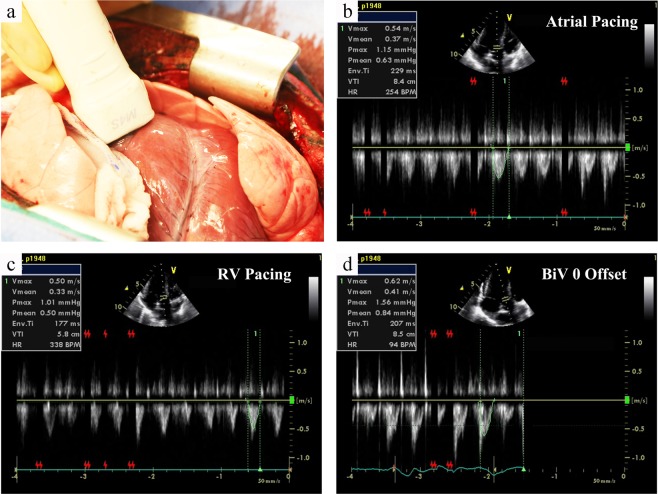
Hemodynamics in the open-chest pacing study. (a) Picture of the hemodynamic response test. Pulse wave Doppler images for (b) atrial pacing, (c) RV pacing and (d) BiV pacing with zero offset. The corresponding VTIs are calculated.
